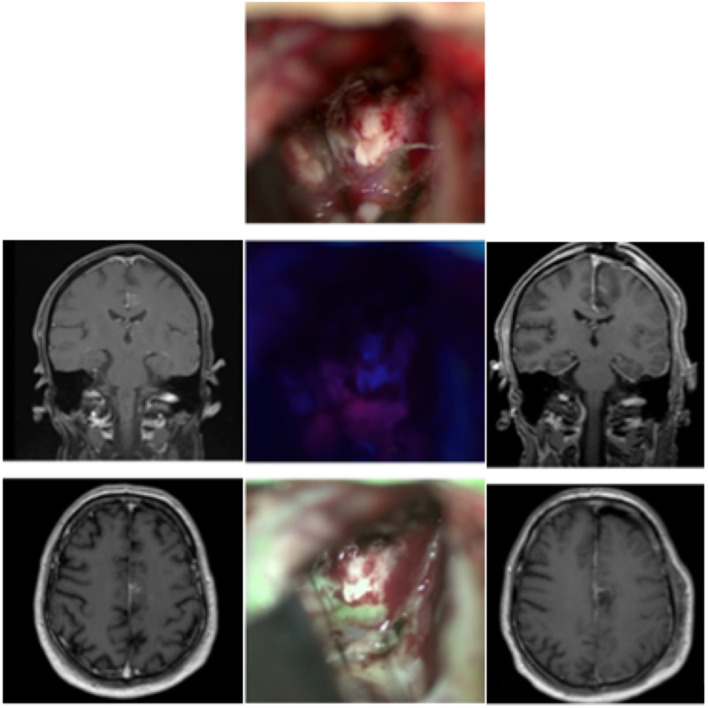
Anaplastic astrocytoma in a 65-year-old patient. The left side shows the preoperative MRI (contrast-enhanced), while the right column shows the early 48 h-postoperative MRI. In the middle column the operative cavity shows the field under white light, below it under blue light, and at the bottom the fusion of both images in the MFL-mode.
